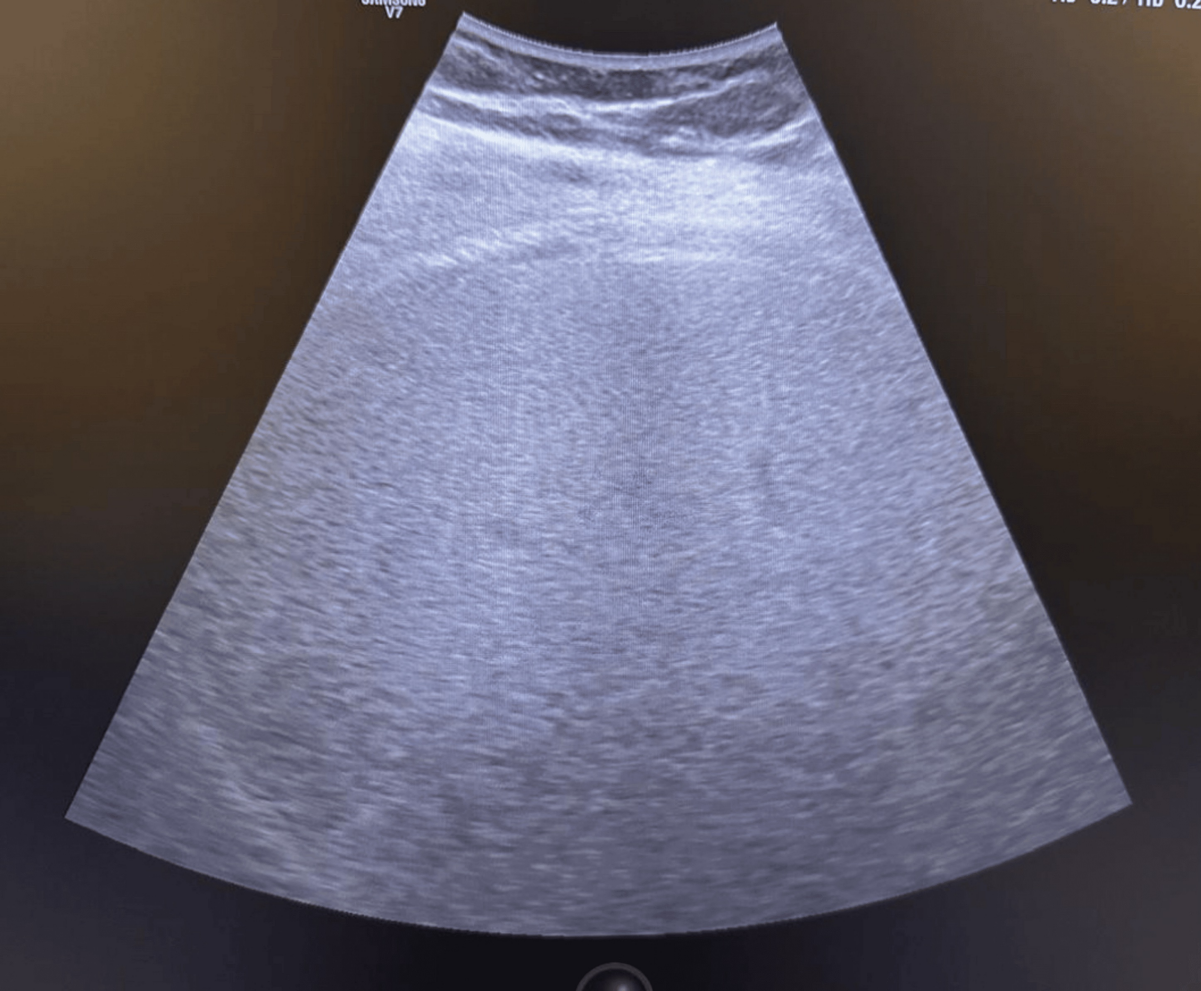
Ultrasound showing grade 2 fatty liver
